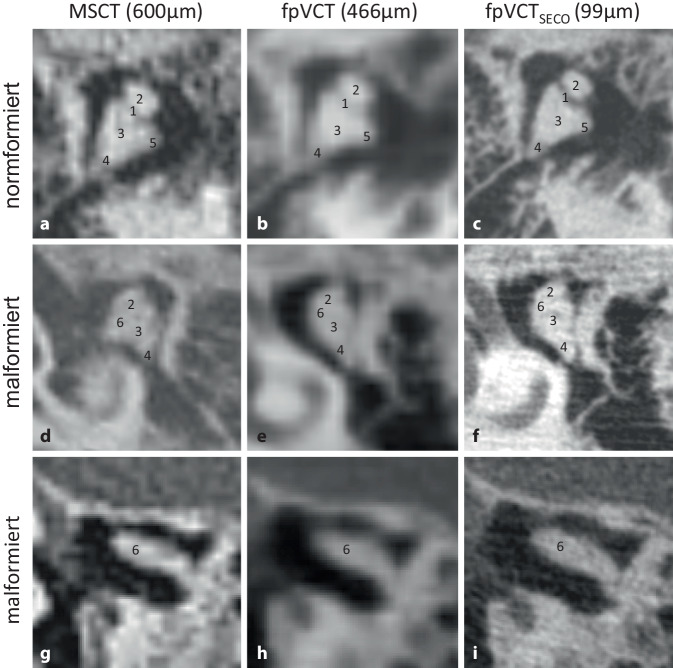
Darstellung des Malleus-Incus-Komplexes mit verschiedenen Bildgebungsmodalitäten und -einstellungen. Ein repräsentatives Bild von MSCT (600 µm Schichtdicke) ist in a, von fpVCT (466 µm Schichtdicke) in b und von fpVCT_SECO_ (99 µm Schichtdicke) in c gezeigt. d–f zeigen den Malleus-Incus-Komplex mit leichten Fehlbildungen, g–i mit schweren Fehlbildungen. Die identifizierten Substrukturen des Malleus-Incus-Komplexes waren *1* Art. incudomalleolaris, *2* Caput mallei, *3* Corpus incudis, *4* Crus breve incudis, *5* Crus longum incudis, *6* verschmolzenes Art. incudomalleolaris. *MSCT* Multislice-Computertomographie, *fpVCT „*flat-panel-volume computed tomography“, *fpVCT*_*SECO*_ sekundäre Rekonstruktionen der fpVCT
